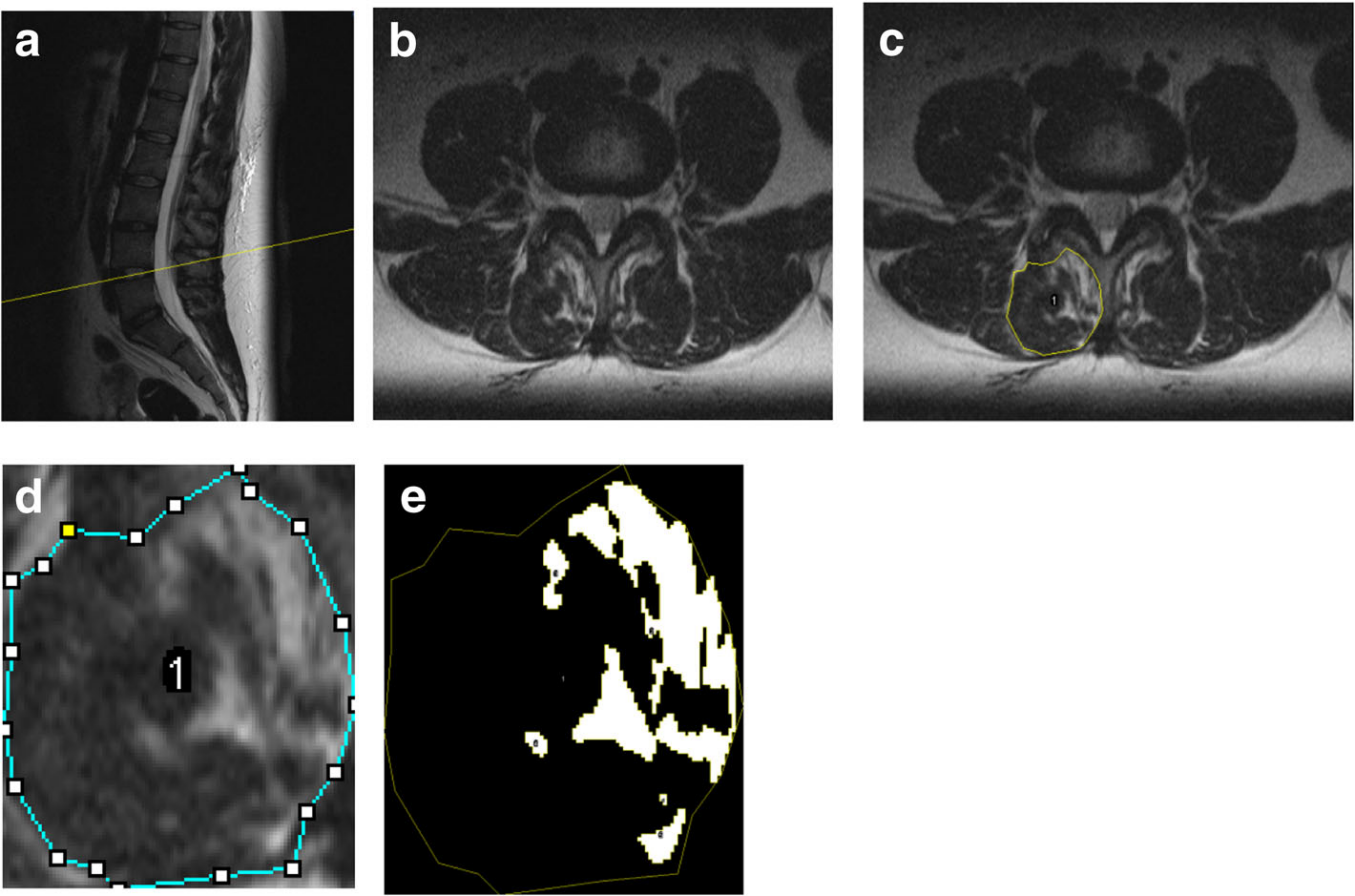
Sagittal T2-weighted images (2a), with a selection of transverse to L4-L5 intervertebral disc, (2b), with a polygonal circumference measurement of the lumbar multifidus (2c), cropping image (2d) and automatic segmentation (2e)
